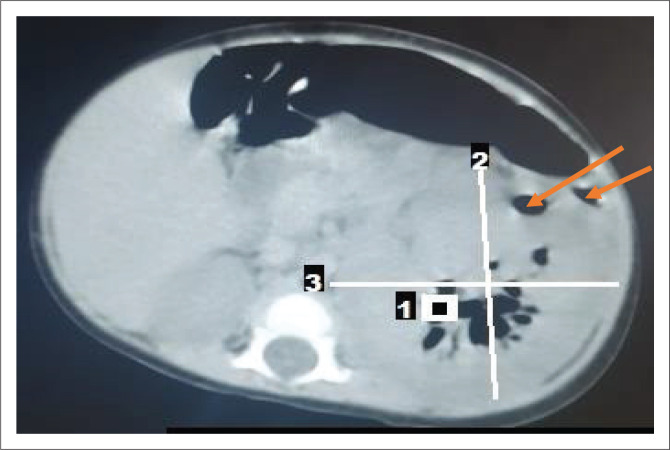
Non–contrast-enhanced computed tomogram of an emphysematous pyelonephritis patient at the Usmanu Danfodiyo University Teaching Hospital, Sokoto, Nigeria, November 2019. Figure shows left renomegaly with multiple air density area within the parenchyma and subcapsular region (orange arrows).
